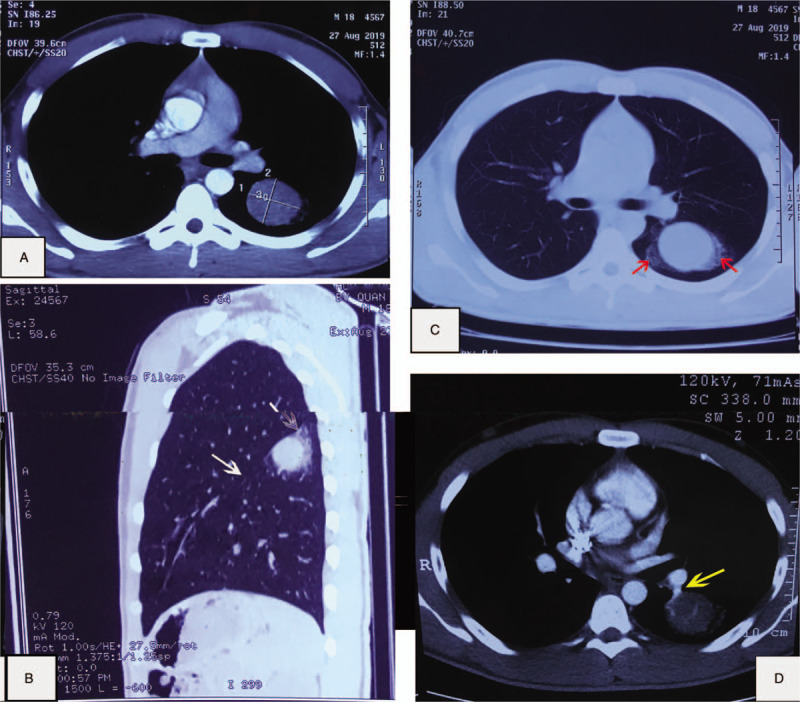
(A and B) A round lesion located in the lower left lobe in thorax CT, below the left greater fissure (white arrows). (C) A mass with surrounding ground-glass opacity (red arrows), defined as the “halo sign.” (D) CT performed 1 mo later revealed that the size had not changed. An obviously enhanced, engorged vascular structure (a yellow arrow) adjacent to the lesion. It was defined as the “overlying vessel sign.” CT = computed tomography.
